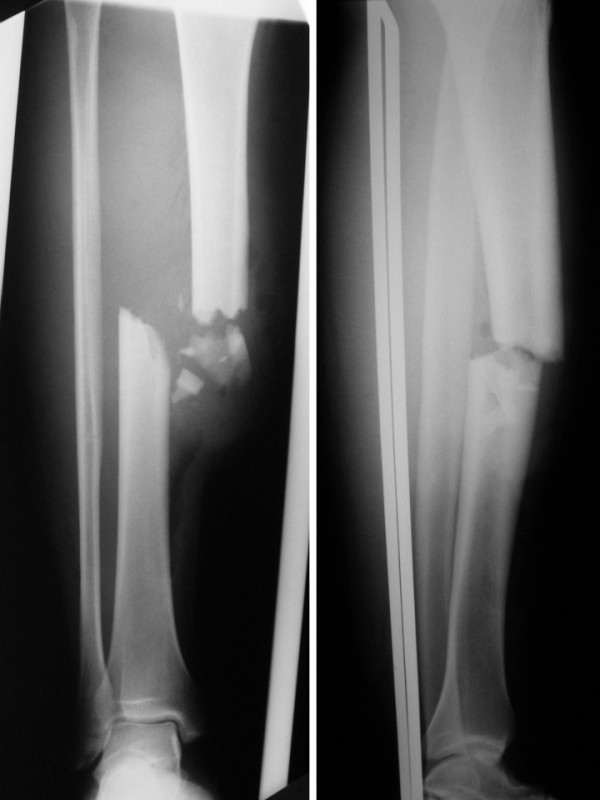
Anteroposterior and Lateral X-ray. The open shaft comminuted tibial fracture.
